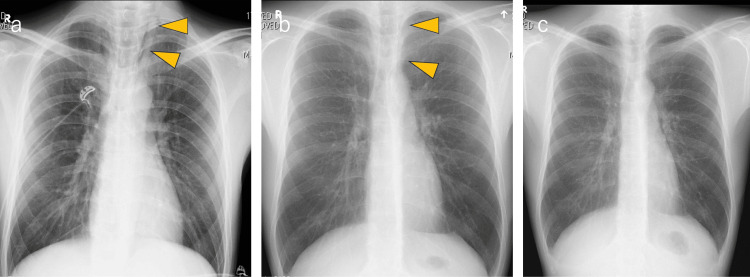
Pneumomediastinum on chest X-rays The spontaneous pneumomediastinum (arrowheads) gradually disappeared from day one (a) to day two (b) and completely disappeared by day nine (c).
